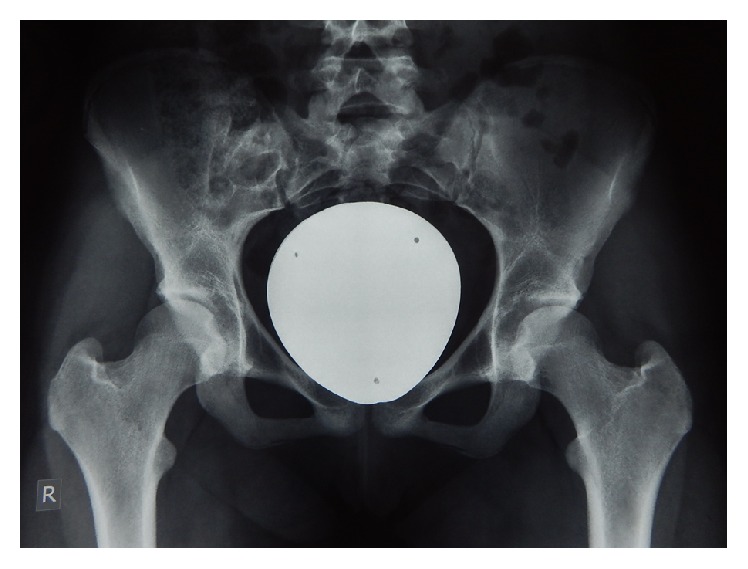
Plain radiograph of the pelvis, initially erroneously diagnosed as normal. Retrospectively, asymmetry of the SIJ is observed which was originally misdiagnosed as bowel gas.
